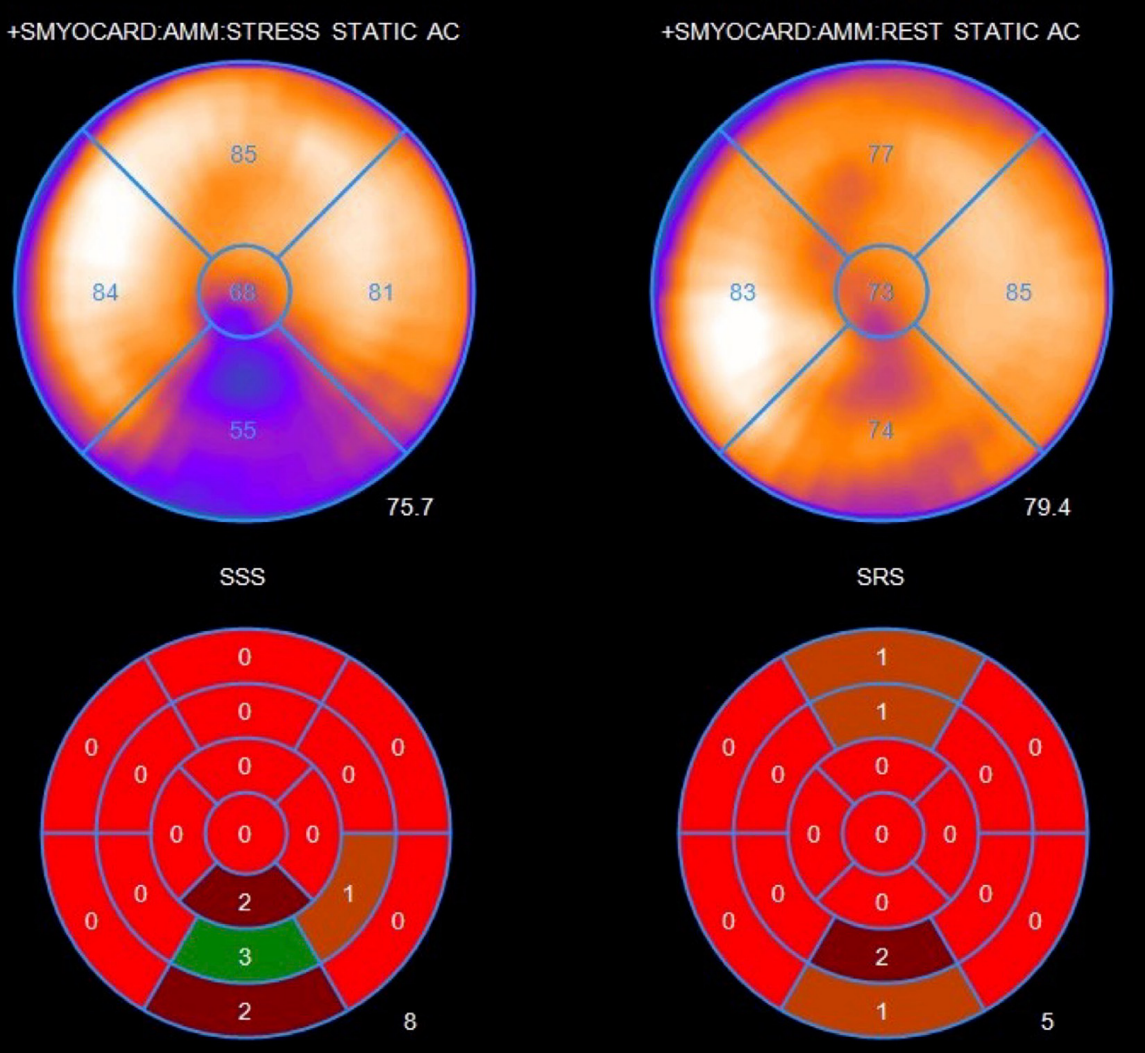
Post-Discharge Cardiac PET-CT Stress Test Postdischarge positron emission tomography-computed tomography (stress and rest) revealed medium-sized, severely dense, reversible ischemia involving 18% of the myocardium, extending from the basal to apical inferior wall, with lowest perfusion noted in right coronary artery territory.
